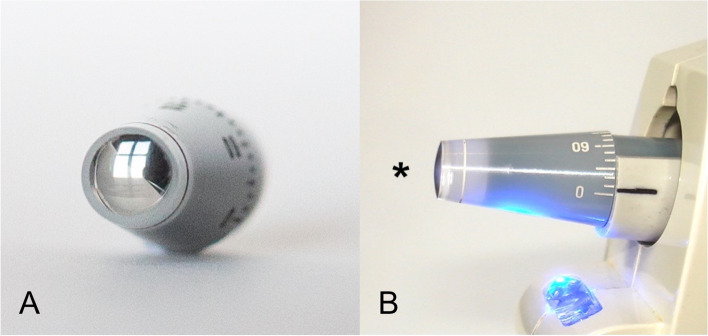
Modified CT applanation tonometer. Observe the convex reflection in the tip (A) and in the side image (B, *) CT tonometer inserted in a handling tonometer device (B)
